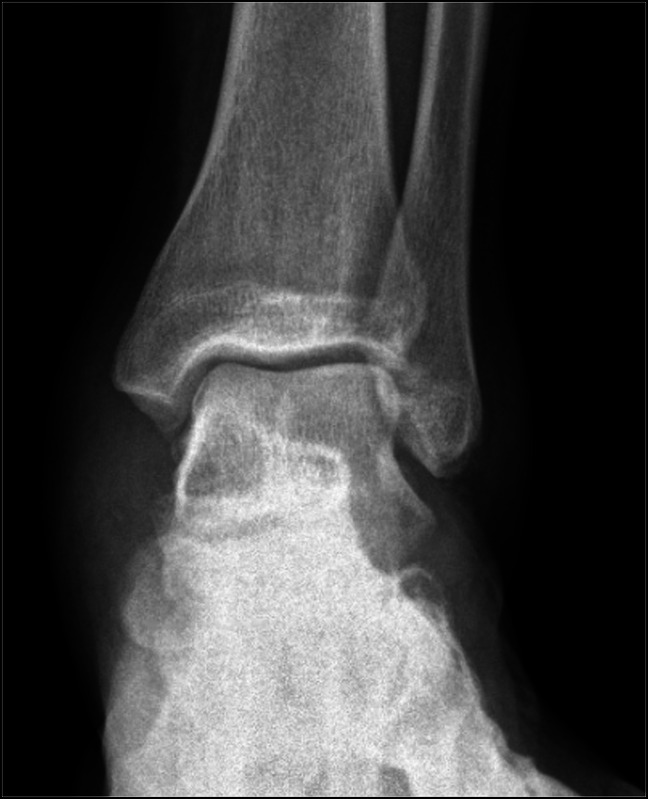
Anteroposterior weight-bearing radiograph image 6 months postreduction without signs of avascular necrosis of the talus.
